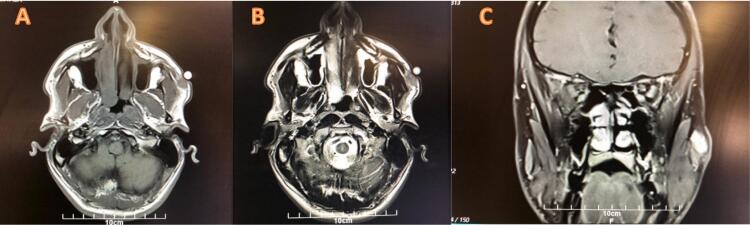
Pre-surgical MRI before surgery reveals a subcutaneous soft tissue lesion in the right zygomatic region, measuring 16*12*15 mm. The lesion exhibits isointense signal relative to muscle on T1- weighted imaging (T1W1) (A) and has intermediate to high signal intensity on T2-weighted imaging (T2W1) (B). The lesion shows vivid enhancement on the post-contrast MRI study (C).
